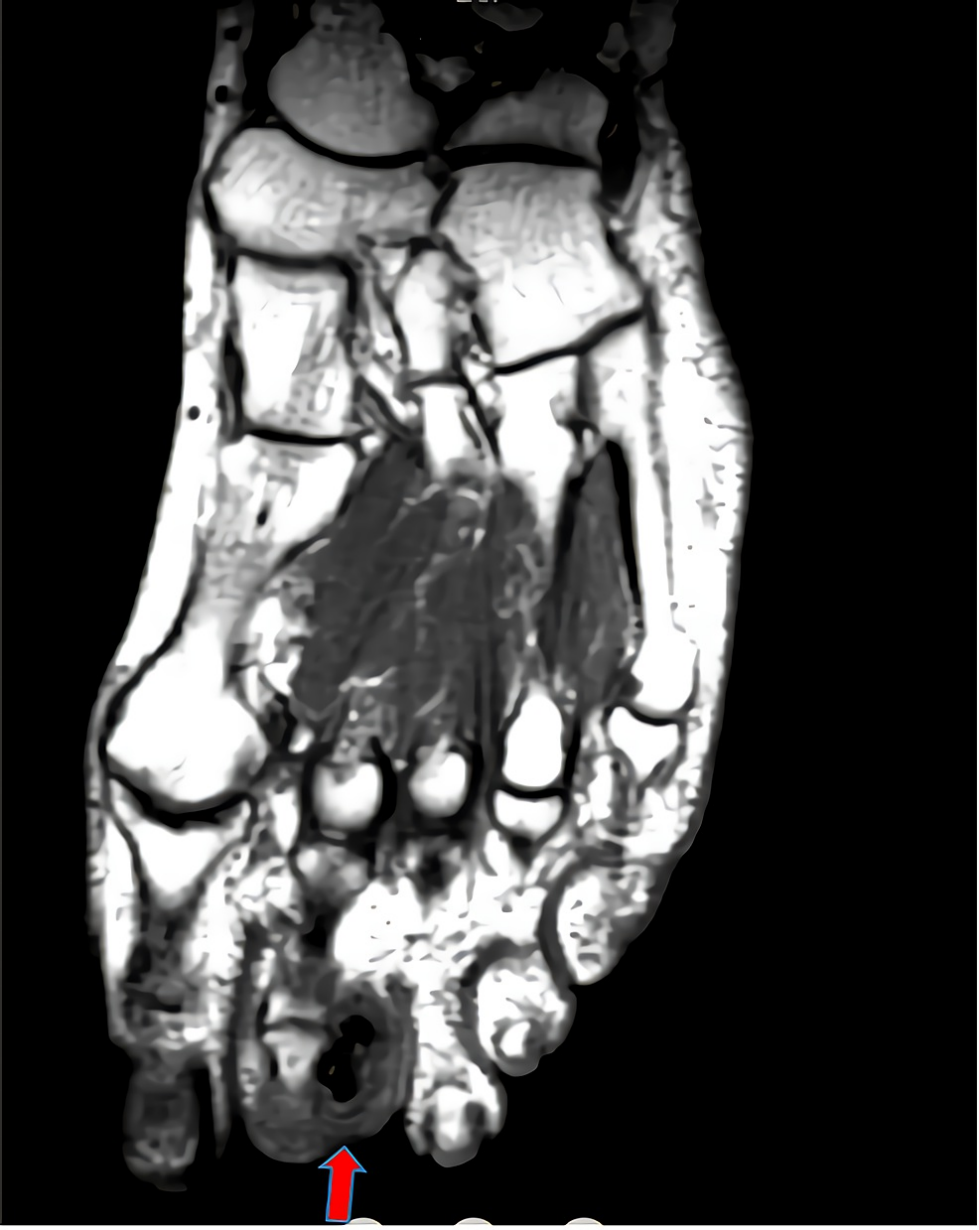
A hypointense lesion was seen on MRI.
